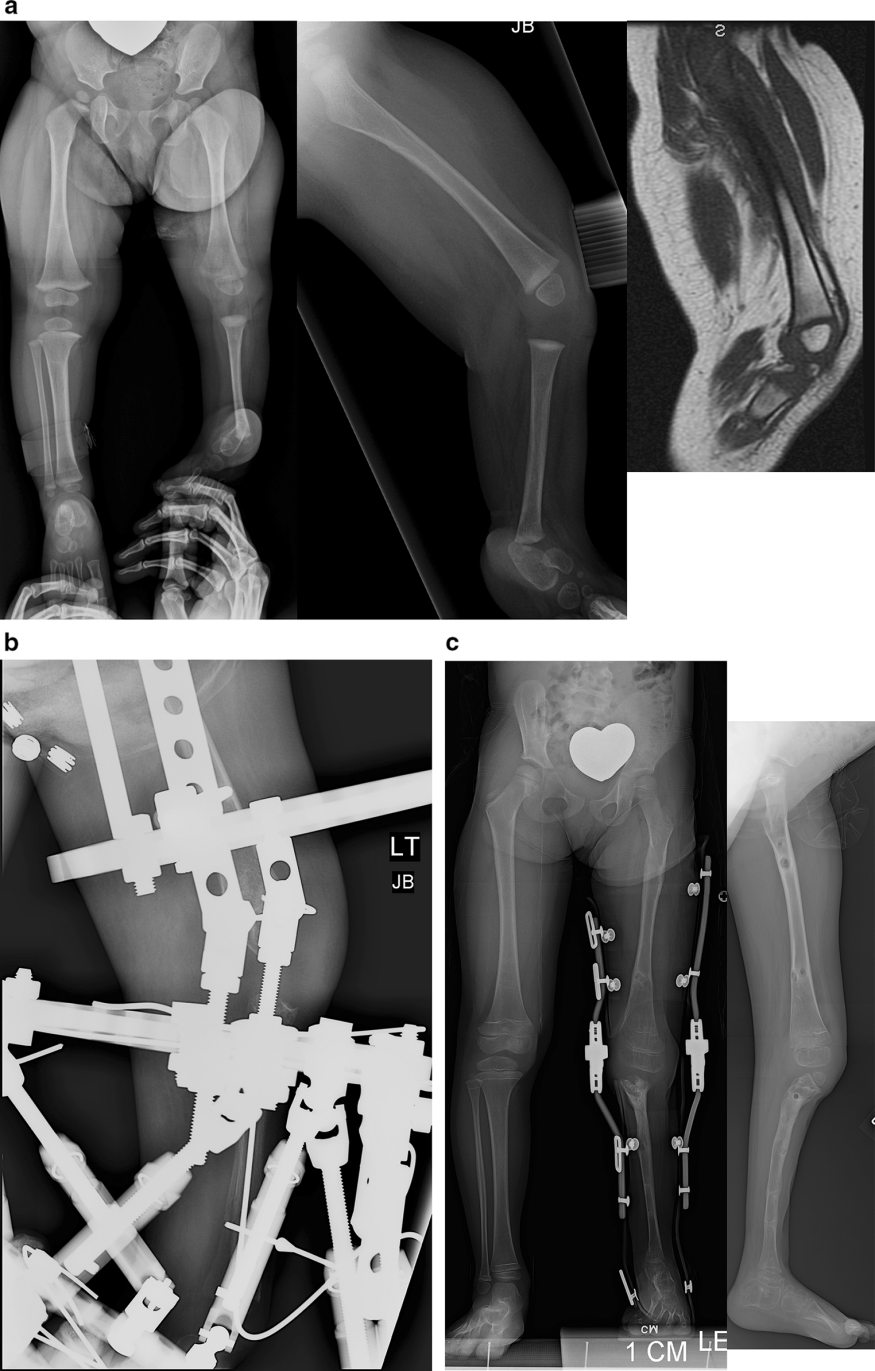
a Antero-posterior (*left*) and lateral (*middle*) radiographs of a 12-month-old girl with Paley type 5B unilateral tibial hemimelia. The fibula is hypertrophied and centered on the femur. The foot is in equinovarus. A sagittal section of the magnetic resonance imaging scan (*right*) shows an absent patella, with a quadriceps muscle extending to the fibula. b Lateral radiograph showing a TSF device with six struts applied between the upper fibula and foot to correct the equino-varus foot deformity and to bring the talus under the end of the fibula. An Ilizarov apparatus on the femur connects to the TSF with hinges at the knee joint. A distraction mechanism is in place to correct the knee contracture while permitting removal of this mechanism to allow physical therapy to move and exercise the knee through the hinges. c Standing AP (*left*) and lateral (*right*) radiographs showing the fibula is centralized, hypertrophied and lengthened. A KAFO brace is used to protect the stability of the knee and promote hypertrophy. The talus is fused to the fibula with the foot in a plantigrade position. The distal and proximal fibular physes are both patent
